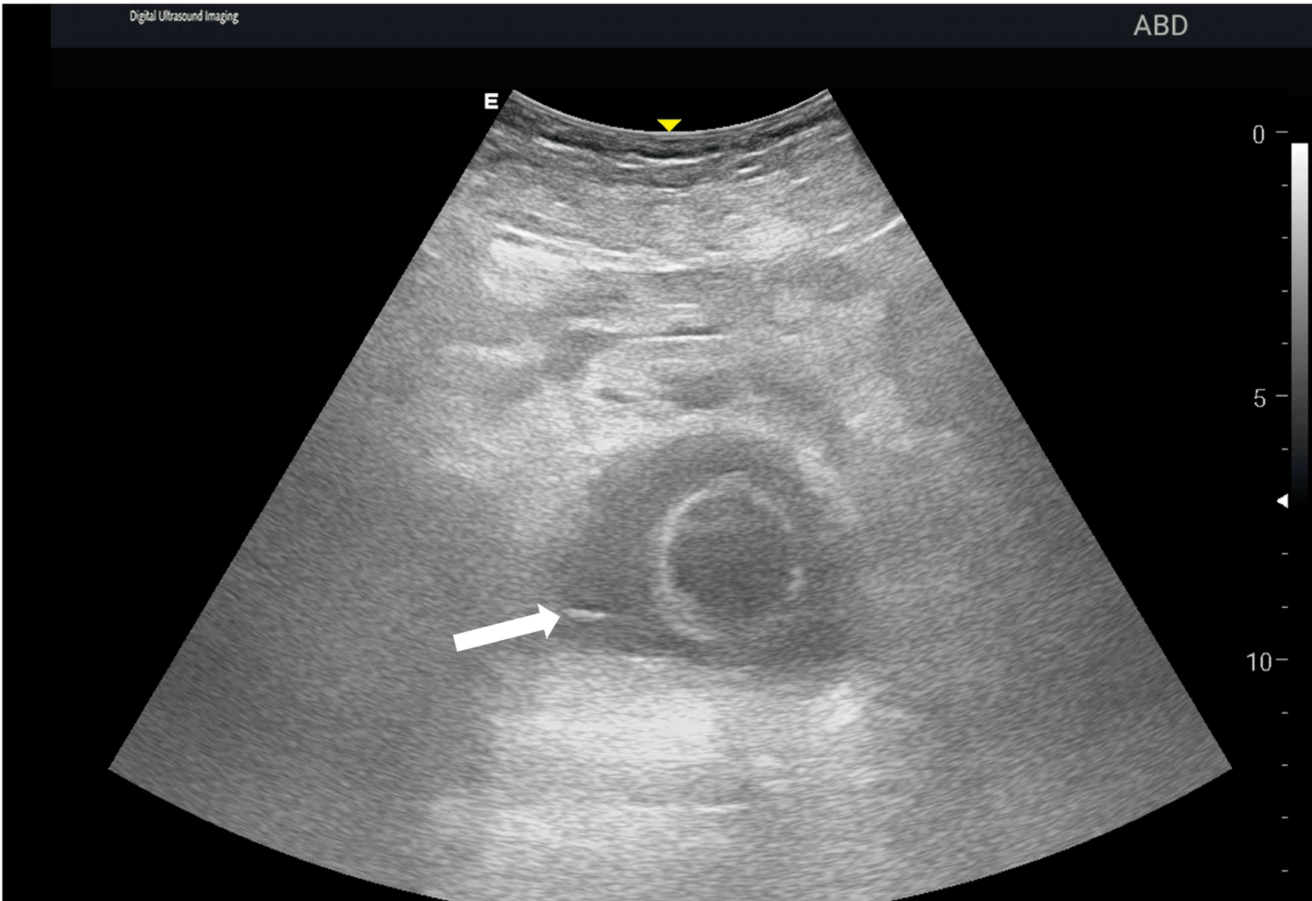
POCUS demonstrating an intravesical foreign body POCUS of the urinary bladder showing a linear echogenic focus within the bladder lumen (white arrow) suggestive of a metallic foreign body. POCUS: point-of-care ultrasound
